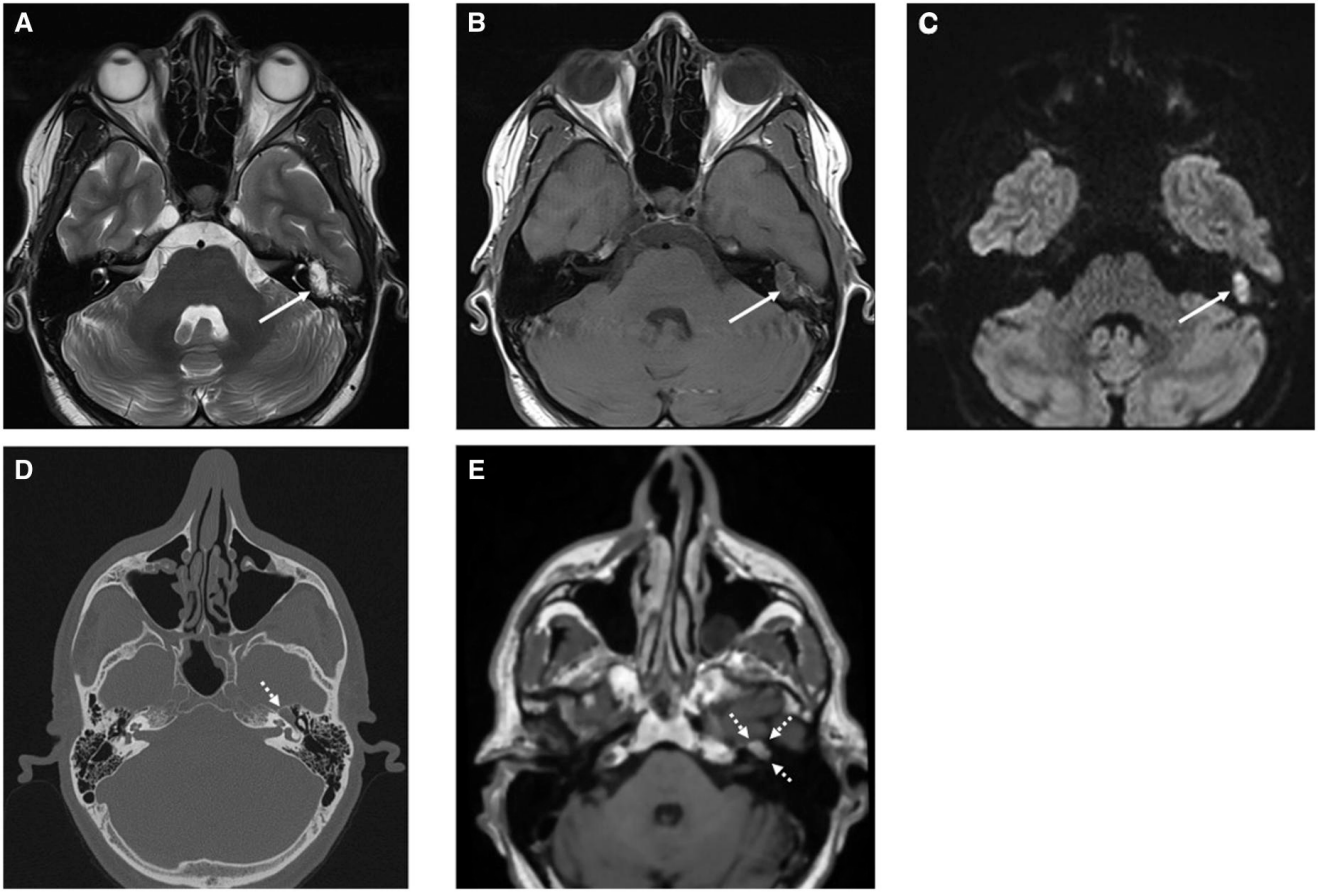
Benign differential diagnoses of the middle ear. (A–C) Acquired cholesteatoma centred on the left mastoid antrum. (A) Axial T2-weighted MRI demonstrates an ovoid and expansile soft tissue lesion filling the antrum. This hyperintense T2-weighted signal (higher than that seen with EMPs) is a key finding as cholesteatomas and EMPs share similar T1-weighted and restriction characteristics, that is, low-intermediate signal on the axial T1-weighted sequence (B) and restricted diffusion (C). In contrast to EMPs, cholesteatomas do not enhance (not shown here). (D, E) Facial nerve schwannoma centred on the left geniculate ganglion. (D) NECT shows lobulated soft tissue (dotted white arrow) expanding the facial nerve canal extending from the labyrinthine segment and involving the geniculate ganglion and anterior portion of the tympanic segment. (E) Non-fat suppressed multiparametric post-contrast T1-weighted MRI demonstrates homogeneous enhancement. There is no restricted diffusion (not shown here).
